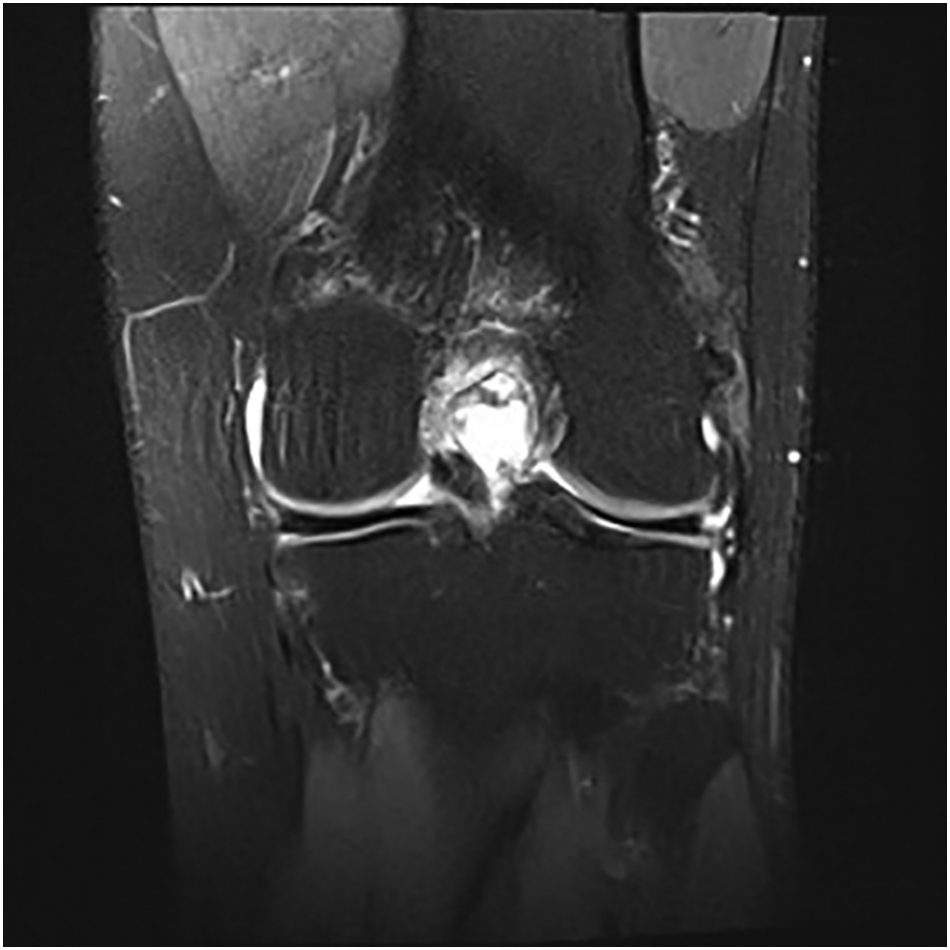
Coronal T3 MRI image (proton density fat saturation) of knee showing large mass filling the notch of the knee and covering the ACL.
